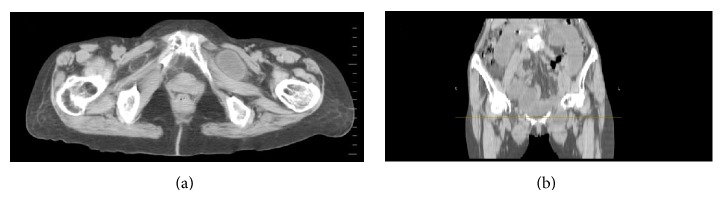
(a) Plain CT scan of inguinal and upper thigh region showing herniated small bowel loop in left obturator canal between pectineus and obturator externus muscles and herniation of mesentery in right obturator canal. (b) Coronal reformatted image at level of obturator canal showing herniated small bowel loop in left obturator canal and herniation of mesentery in right obturator canal.
